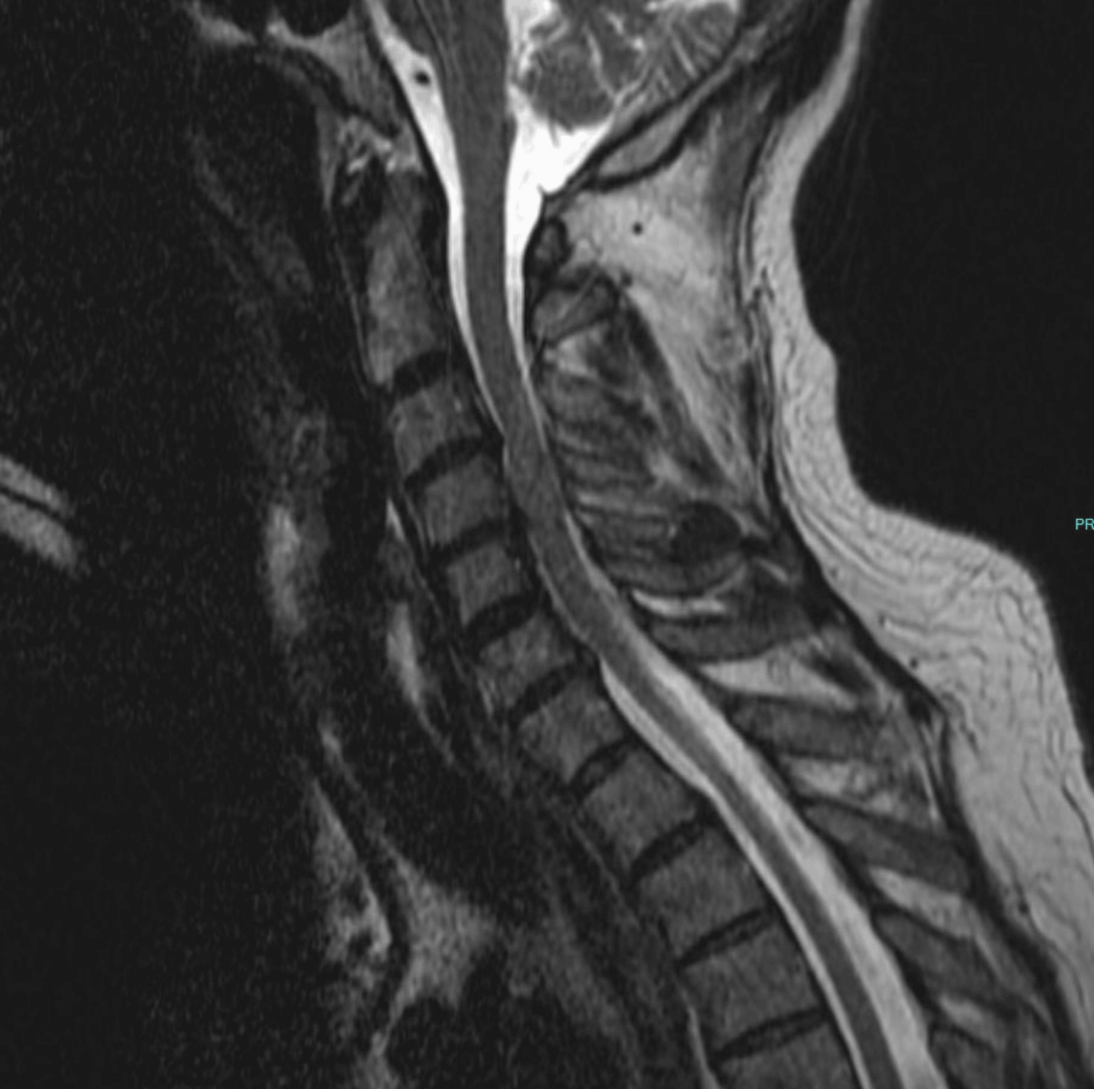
Case E: MRI of cervical spine. There is mild to moderate left neural foraminal disc herniation of the C6-7 level. There is no fracture, spondylolisthesis, or spinal cord mass lesion causing compression.
